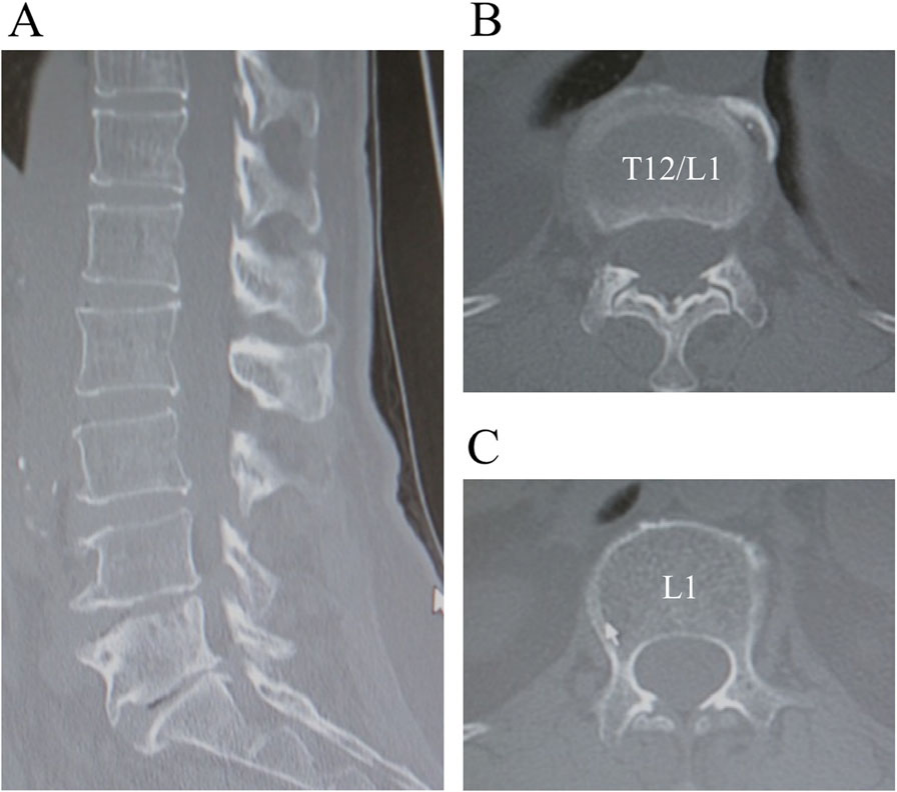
Computed tomography (CT) of thoracolumbar spine. CT of the thoracolumbar spine showing a sagittal, b axial at T12–L1, and c axial at L1 views. There were no specific findings with regard to the tumor, and there was no calcification in the spinal canal
